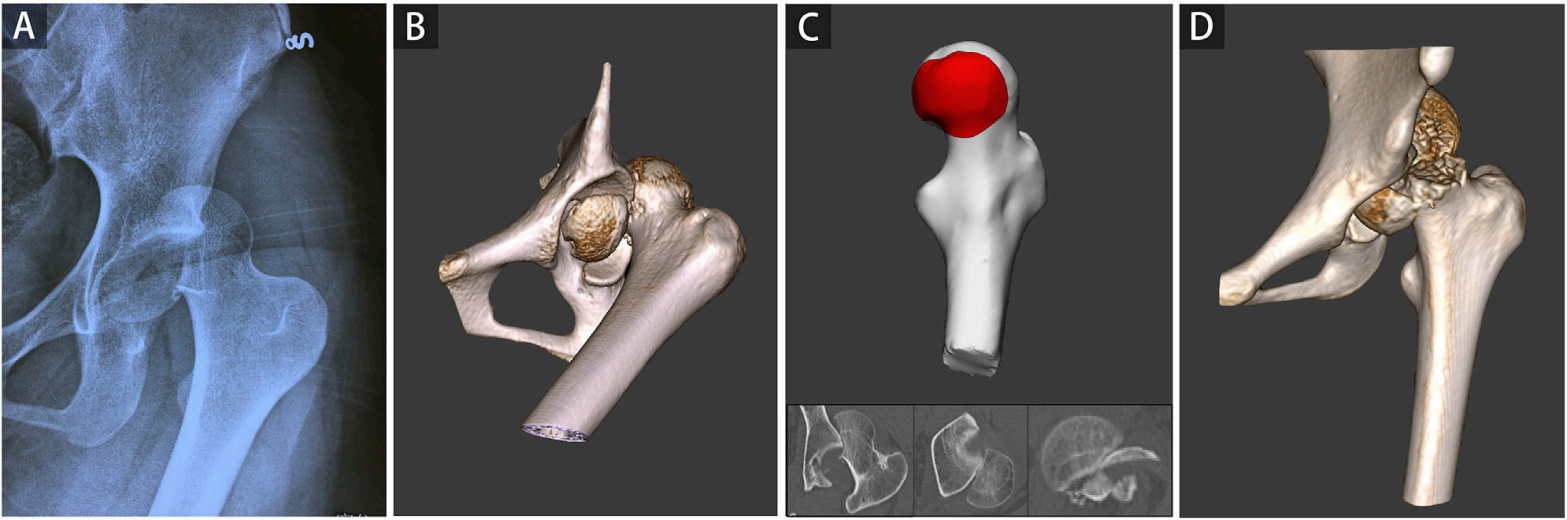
A representative case of iatrogenic femoral neck fracture during closed reduction was shown. A 16-year-old girl involved in a severe traffic accident was diagnosed with femoral head fracture based on X-ray (A) and CT (B). The average percentage of maximum defect length across three planes of 92.80% in the 2D injury model and the percentage of fracture area of 38.33% in the 3D injury model were determined (C). A refracture of the femoral neck occurred during closed reduction (D).
